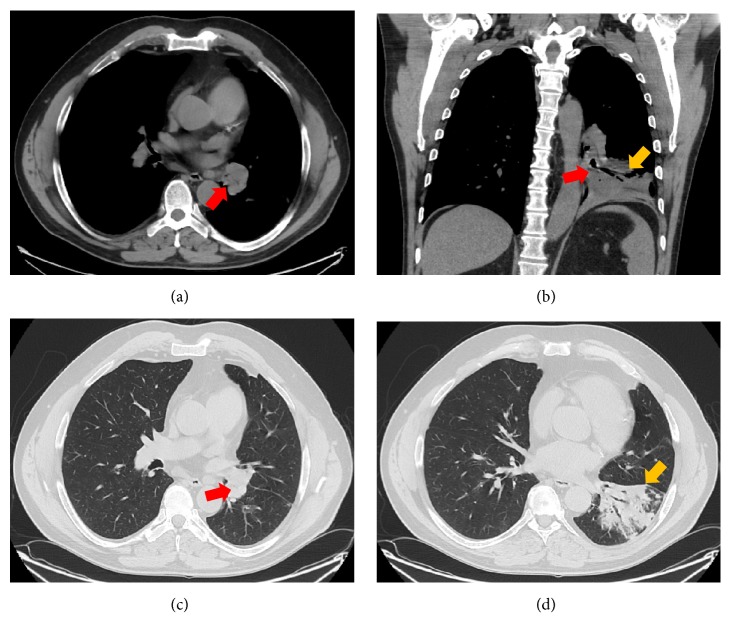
Computed tomogram (CT) of the chest (mediastinal window) in horizontal view (a) and coronal view (b) showing a narrow left lower lobe (LLL) bronchus (red arrow) and distal postobstructive consolidation (yellow arrow). CT of the chest (lung widow) in horizontal view ((c) and (d)) also depicting the narrowed LLL bronchus (red arrow) with the distal consolidation (yellow arrow).
